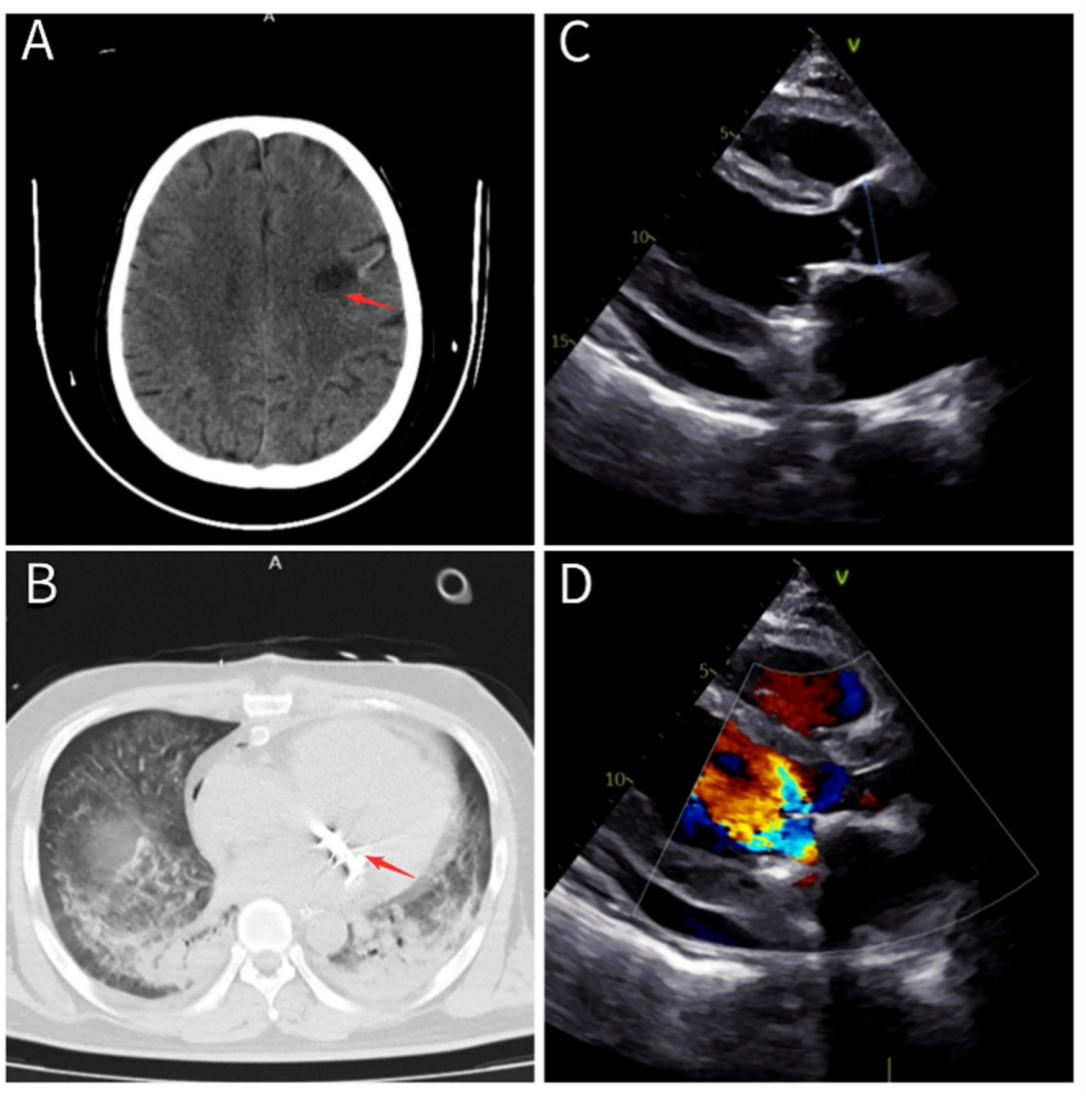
(A) Cranial CT revealing an infarction in the left frontal lobe (red arrow). (B) Chest CT confirming correct placement of the mitral mechanical valve (red arrow). (C,D) Echocardiographic images demonstrating normal function of the mitral mechanical valve with no residual intracardiac masses.
